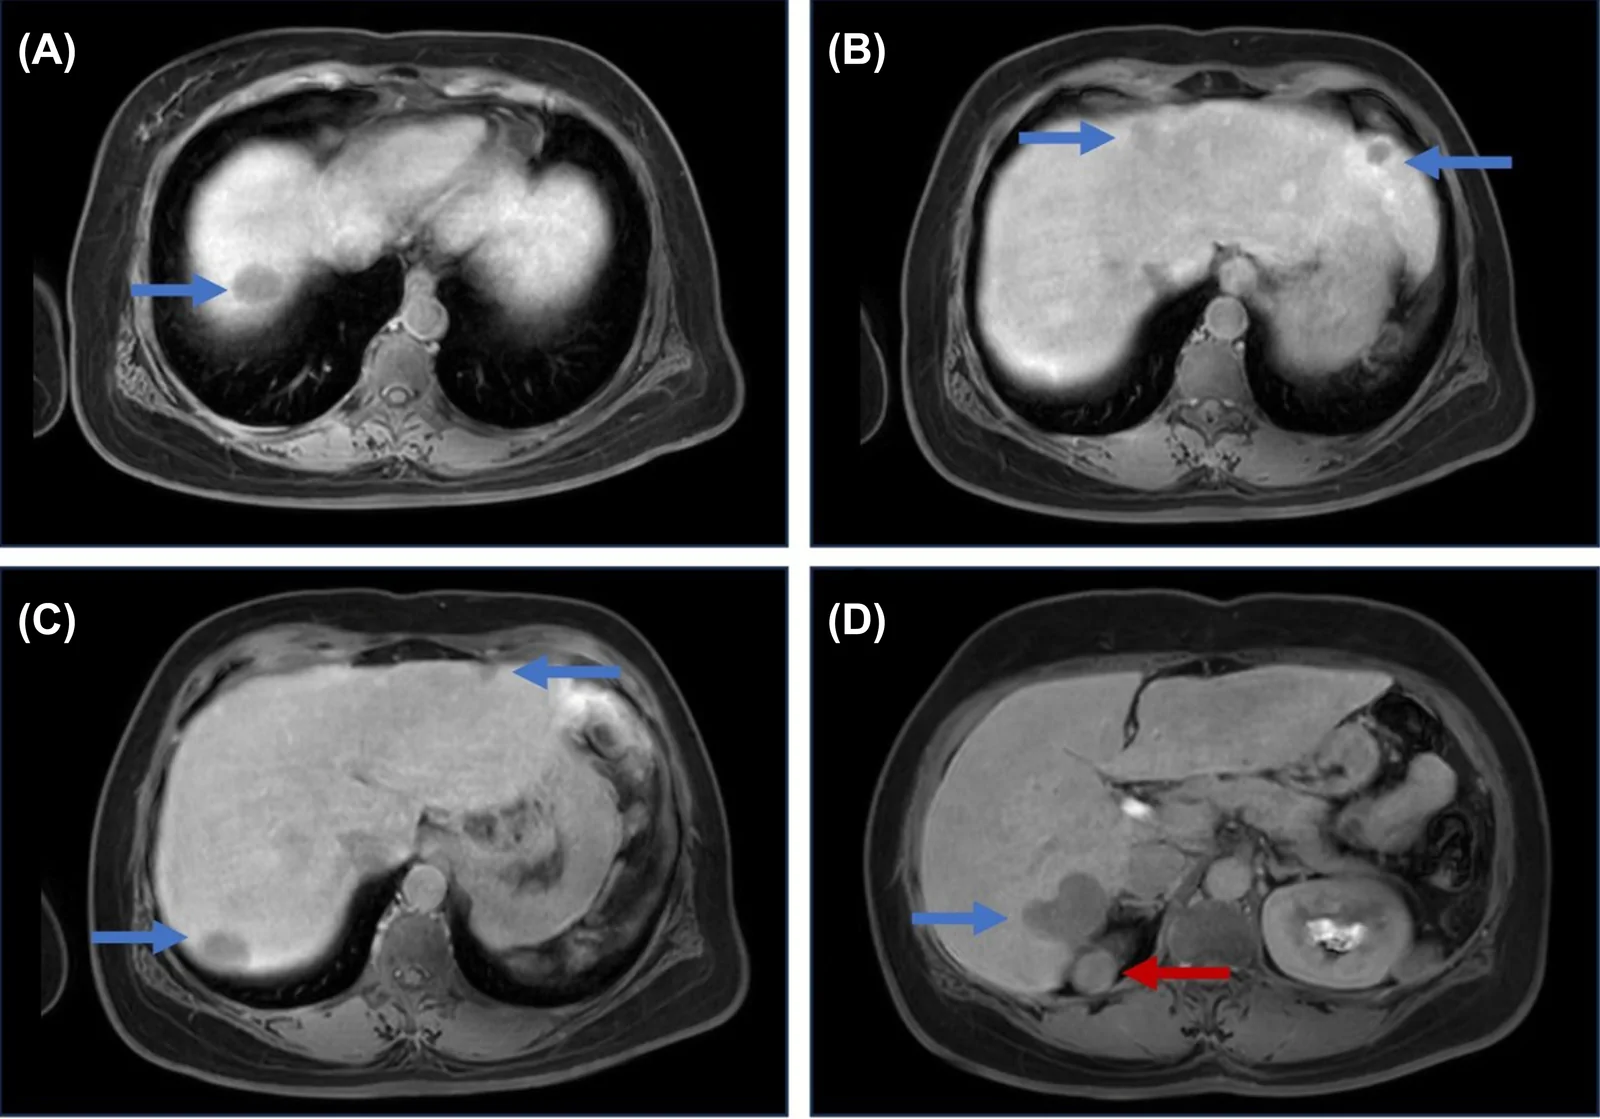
Splenic implant lesions distribution in MRI scans. (A) Intrahepatic splenosis lesion (blue arrow) No.1, (B) Intrahepatic splenosis lesions No.2 and No.3, (C) Intrahepatic splenosis lesions No.4 and No.5, (D) Intrahepatic splenosis lesions No.6 and visceral peritoneum splenic implant lesion (red arrow).
